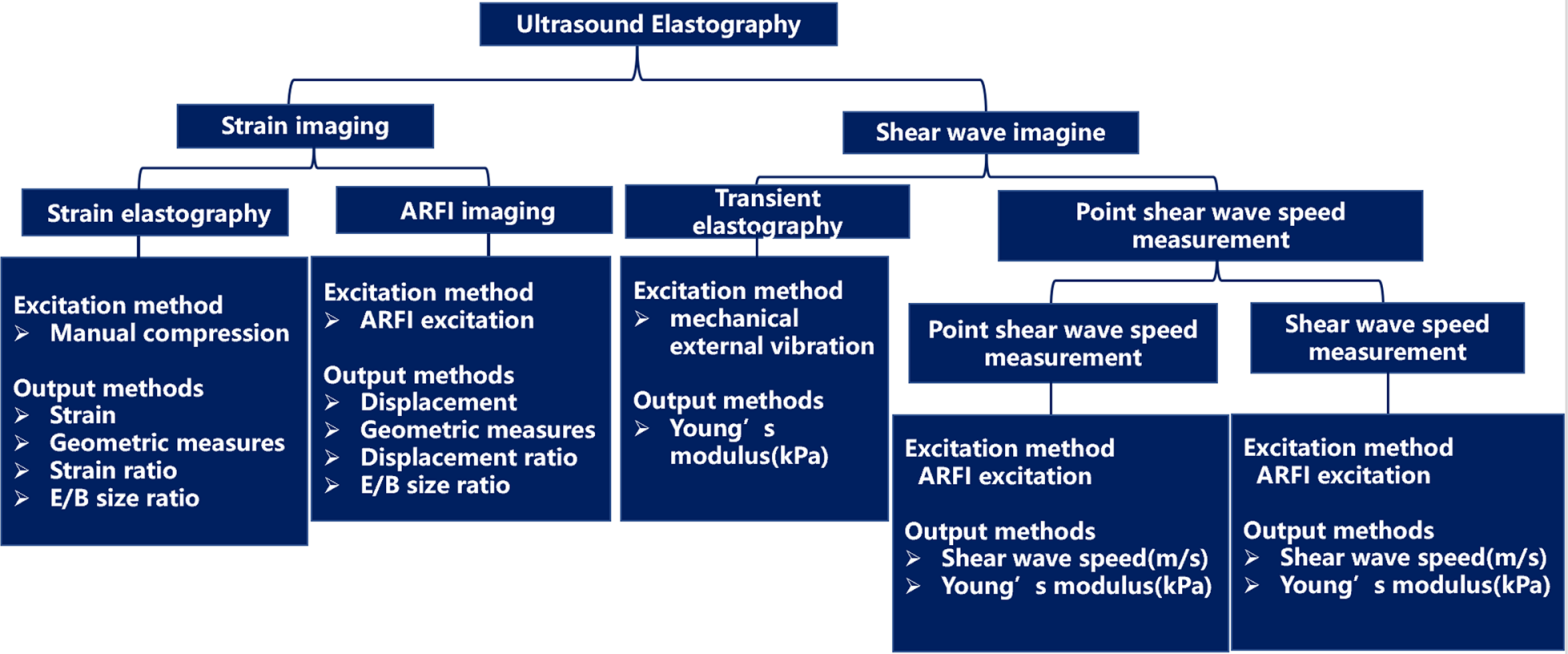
The excitation and output methods for different ultrasound elastography modalities. *ARFI* acoustic radiation force impulse
